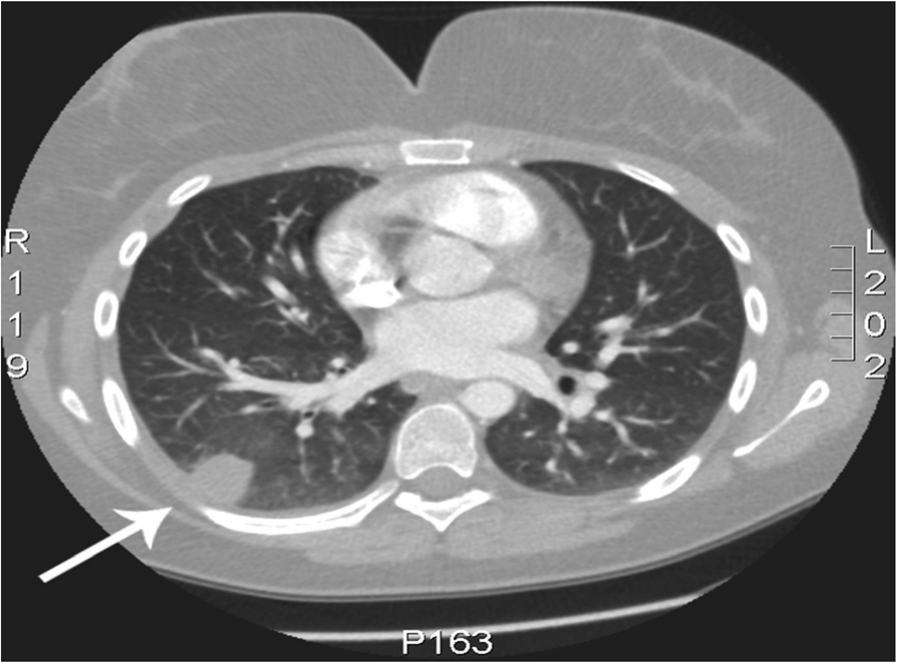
Thoracic CT-scan showing a consolidation in the right inferior lobe (arrow) interpreted as a pulmonary infarction due to pulmonary embolism from the cardiac thrombus
